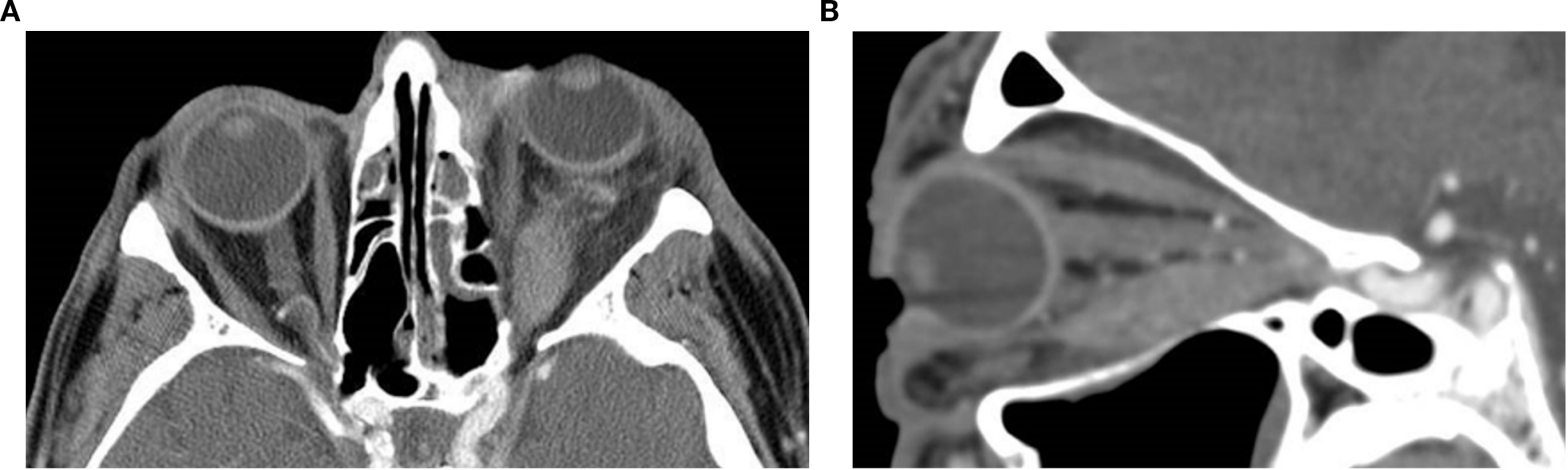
Axial (a) and sagittal oblique reformat (b) post-contrast CT images of the orbits in a 55-year-old male patient with left-sided orbital myositis demonstrate diffuse thickening and enhancement of the inferior rectus muscle with associated moderate axial proptosis. There is associated infiltration of the intraconal and extraconal fat, particularly in the temporal quadrant. The remaining extraocular muscles are unremarkable. The muscle enlargement results in mild medial displacement of the optic nerve complex (not shown), without significant retrobulbar crowding or orbital apex involvement.
